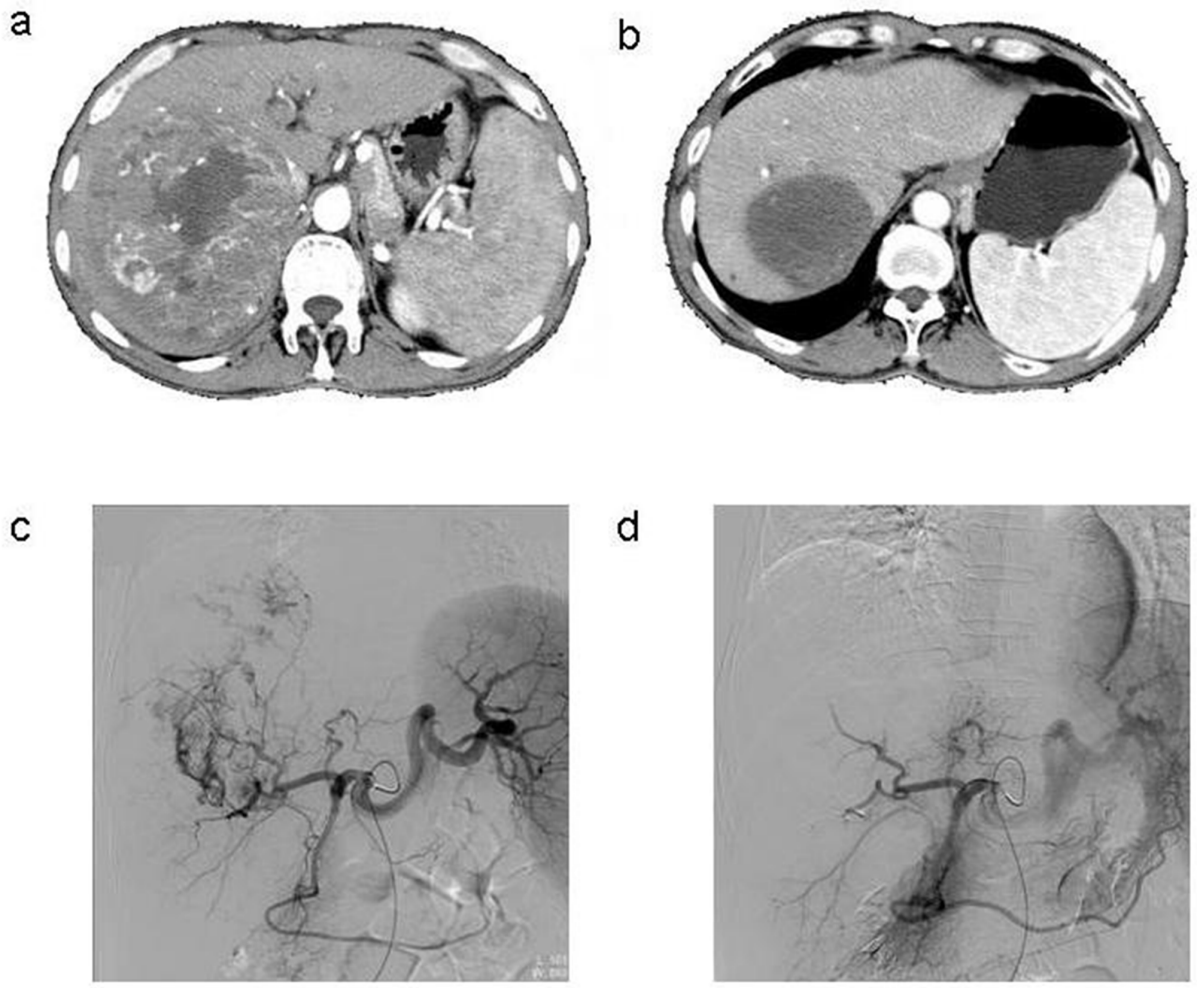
a. Contrast-enhanced CT scan done before the procedure in the patient with HCC, b. Enhanced CT showed complete tumor necrosis 6 months after three TACE sessions, c. Common artery angiography of the patient with HCC during 1^st^ TACE, selective catheterisation of the pathologic branch of right hepatic artery supplying the tumor, d. After chemoembolization angiography with 1^st^ TACE.
